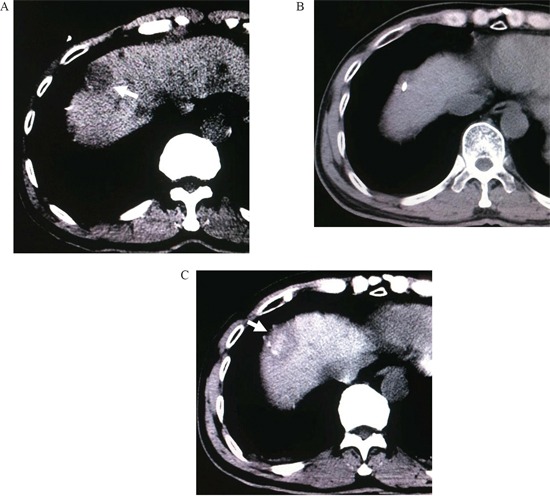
A. CT scan before treatment, a hepatic dome lesion can be found (arrowhead). B. A MW antenna was inserted into the lesion by the established model. C. Complete necrosis of tumor without enhancement after ablation (arrowhead).
